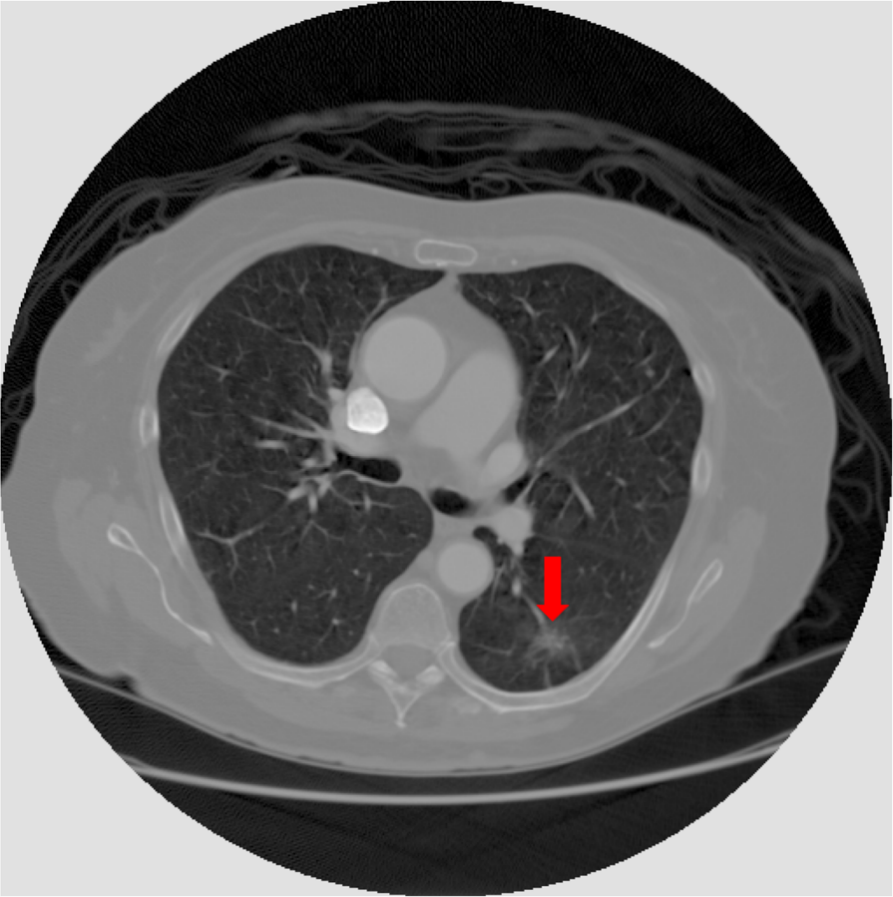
CT image presenting a pulmonary nodule (*red arrow*)
